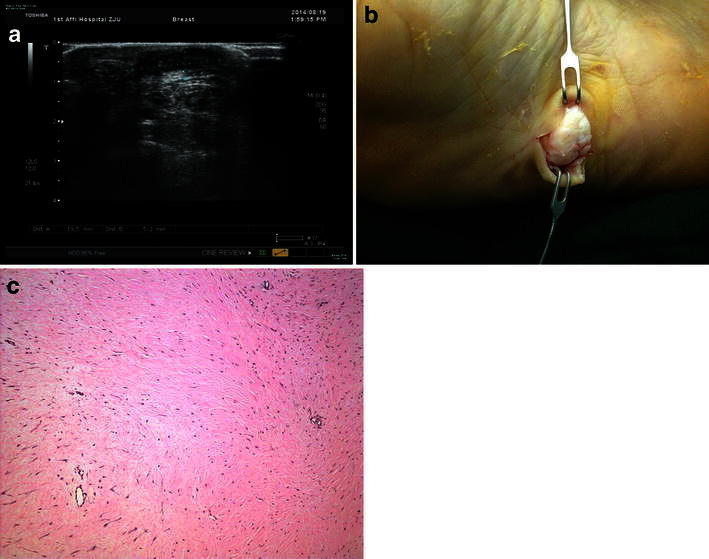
a Ultrasound indicates the lesions were located under superficial layer of the planta before operation. b The tumor was located above the plantar fascia. c For the superficial type, the vogue lobulated lesion was arranged. The lesion constituted discrete fibroblast, the confined lacuna vasorum and red collagen fibers. Fibroblast had the shape of short shuttle, the partial cells were rich with bundles of spindle-shaped arrangement (magnification ×100)
